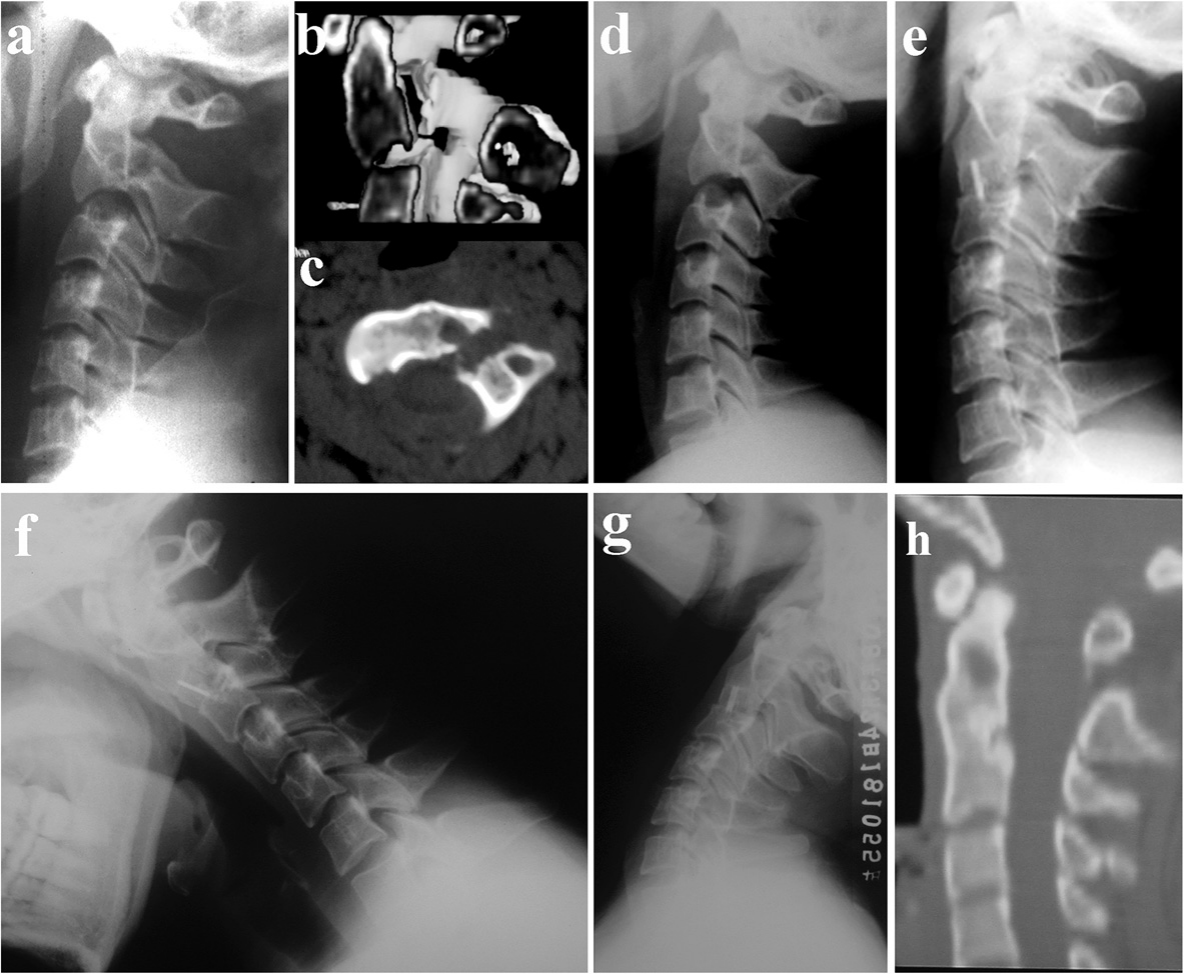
Images of a 38-year-old male patient. (a, b) Preoperative lateral X-ray and CT scans showing a type IIA Hangman’s fracture with severe angulation. (c) CT with axial section showing a bone cyst in the vertebral body of C2. (d) Some degree of reducing was accomplished during skull traction for 3 days. (e) Three-month postoperative lateral X-ray after ACDF with PEEK cage showing adequate reduction and bony fusion. (f, g) Twenty-four-month flexion/extension lateral X-rays showing no range of motion at the fusion site. (h) CT with sagittal reconstruction showing solid fusion and fracture healing. *ACDF* anterior C2/3 discectomy and interbody fusion. *PEEK* polyetheretherketone.
